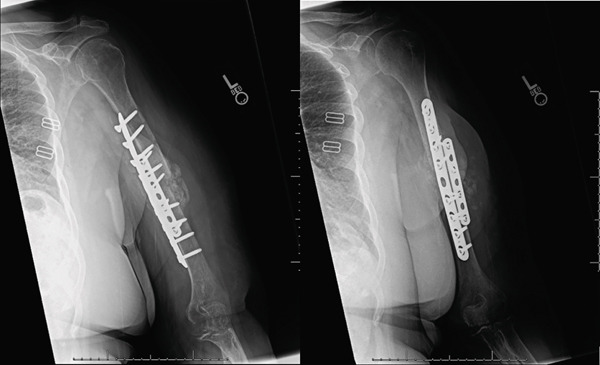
Radiographs at 5 weeks postoperatively demonstrate improved alignment and callus formation. The plate and screws are not pulling off the plate any further.
